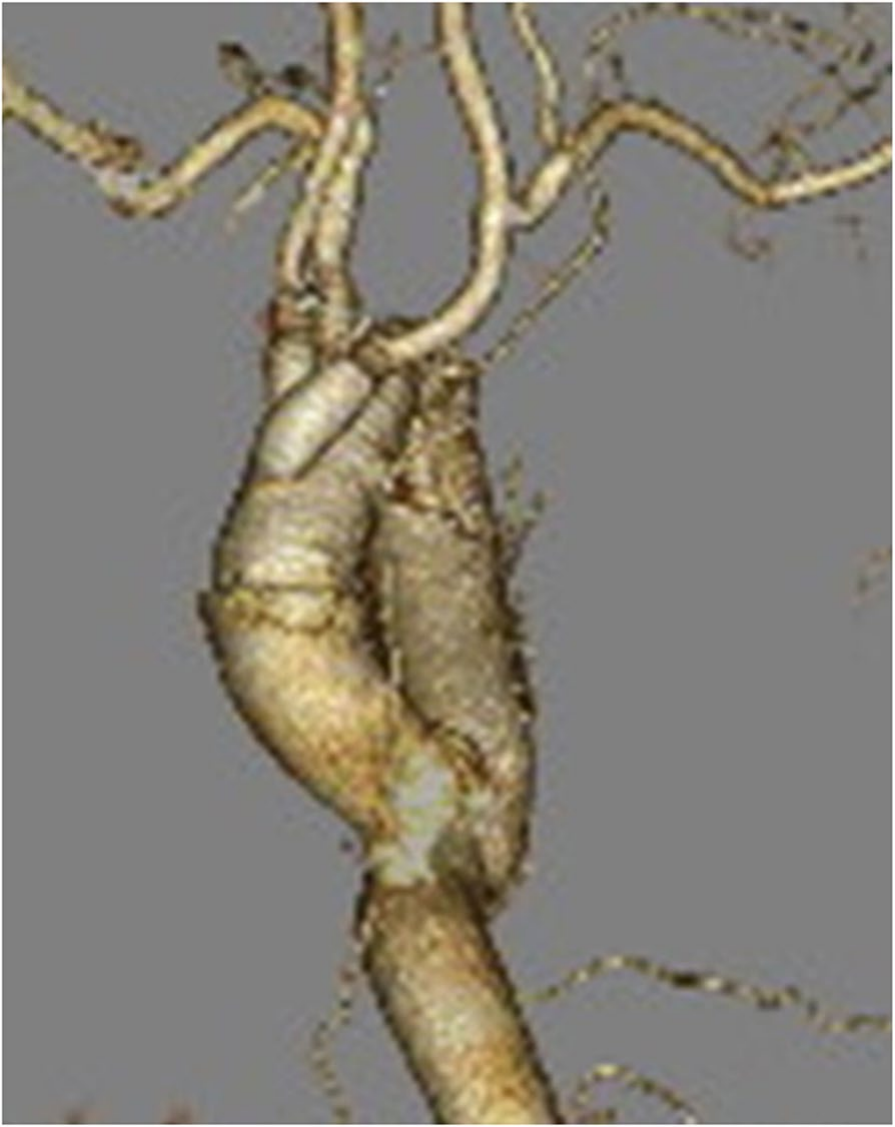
Postoperative computed tomography image
